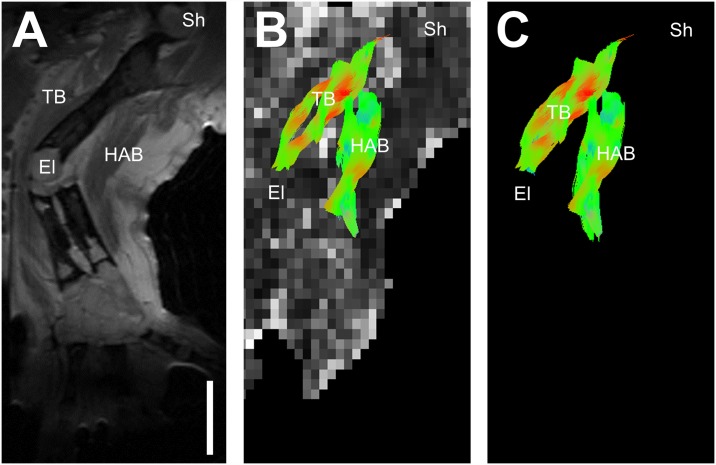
Diffusion tractography images of an adult axolotl’s right upper arm and its positioning for image acquisition. A: A T2-weighted image showing anatomical structures. B: Reconstructed fiber pathways by tractography I overlaid on the fractional anisotropy map. C: Reconstructed fiber pathways by tractography I of the entire right upper arm. Color has been assigned to indicate the direction of the local fibers; red: left-right, blue: back-front, green: up-down directions. Sh = shoulder, HAB = humeroantebrachialis, TB = triceps brachii, El = elbow. Scale bar = 5 mm.
